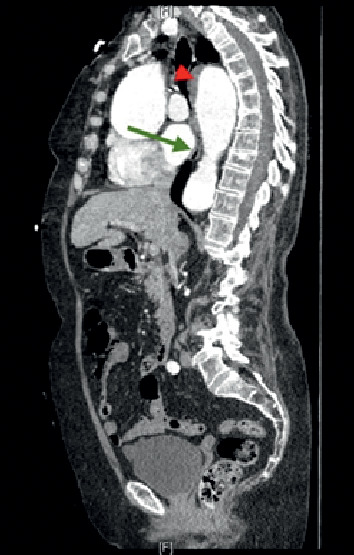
Sagittal section of CT angiogram of the chest showing thoracic aorta aneurysm of approx. 5.5 cm size causing extrinsic compression at midesophageal level (green arrow) along with intramural thrombus in the thoracic aorta (red arrowhead).
